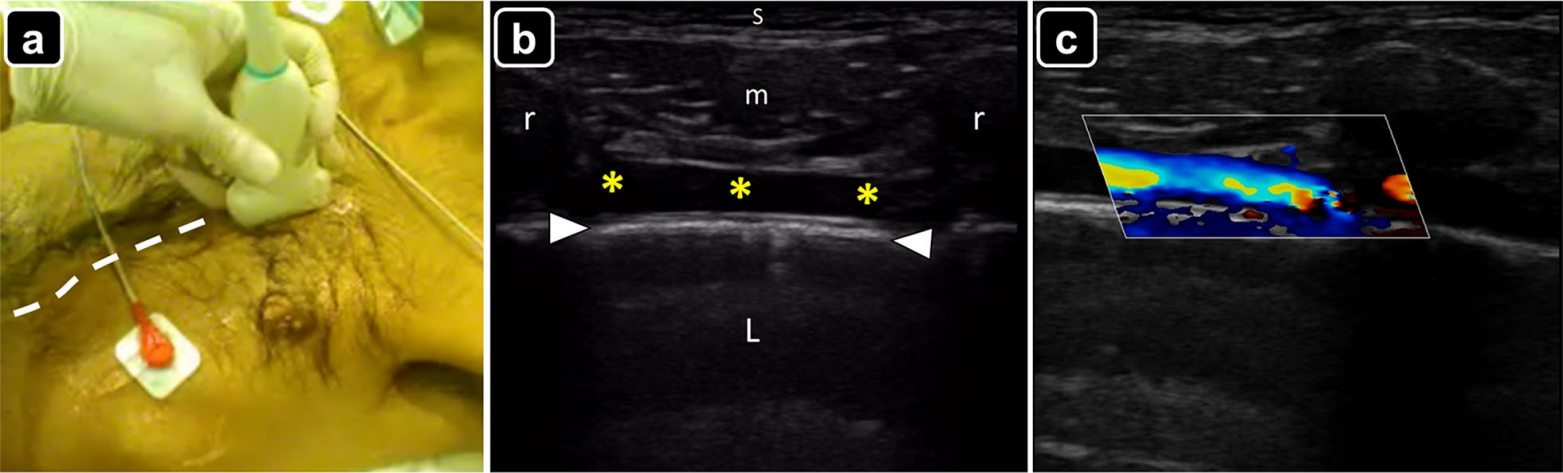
a Technique for localization of the internal thoracic vessels (dotted line) using a linear probe along the left parasternal line. b Identification of the internal thoracic vessels (asterisks) on ultrasound; *s* skin and subcutaneous tissue; *r* ribs; *arrowheads* pleural line; *L* lung. c Color Doppler showing blood flow in the internal thoracic vessels
